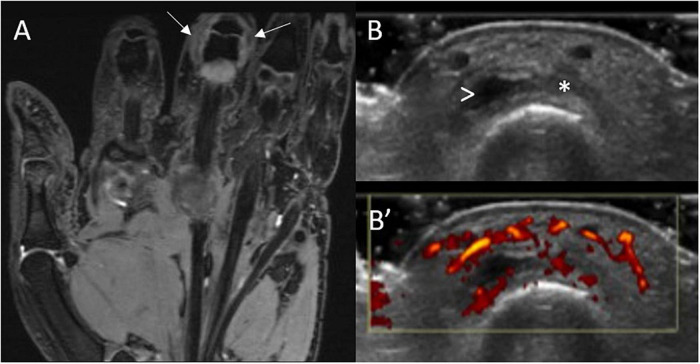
MRI and US sub-clinical synovitis in a patient with CSA and high titre ACPA. MRI (A) synovitis (white arrows) of the 3rd PIP joint in an individual at-risk of RA with corresponding gray-scale (B) and power Doppler (B’) US scans. This individual had clinically suspect arthralgia and positive anti-CCP antibodies (high titre) and rheumatoid factor. These images were obtained 6 weeks before progression to RA. Asterisk indicates synovial hypertrophy, while the arrowhead a small joint effusion.
